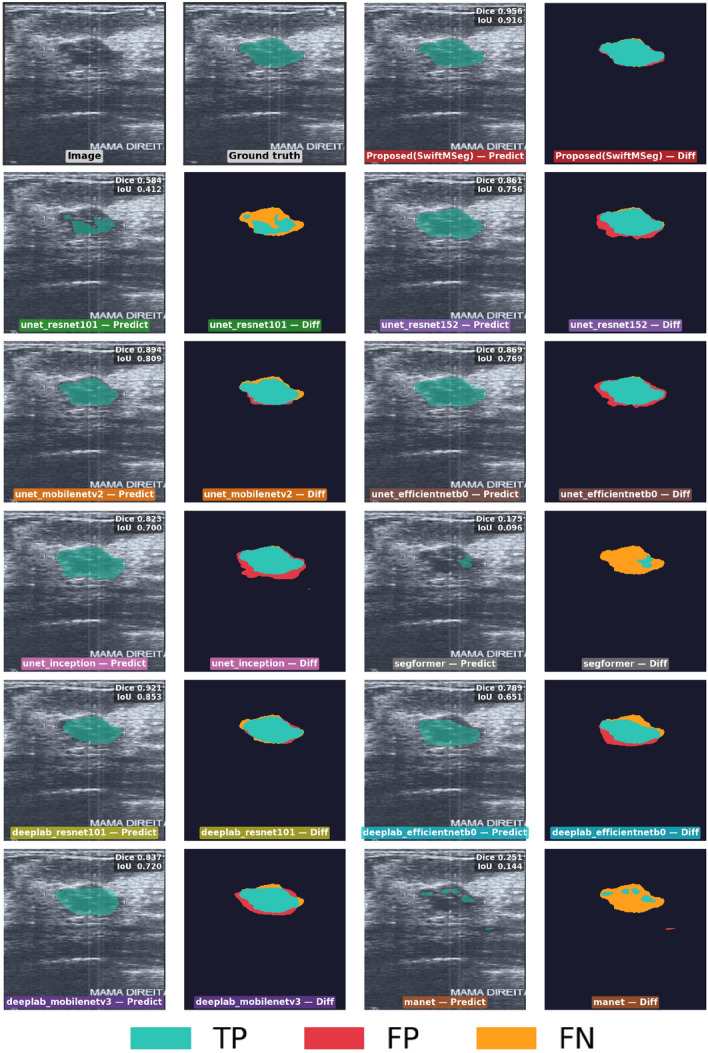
Breast tumor segmentation in ultrasound image (BUS-BRA^48^) using different models (*TP* true positive, *FP* false positive and *FN* false negative).
